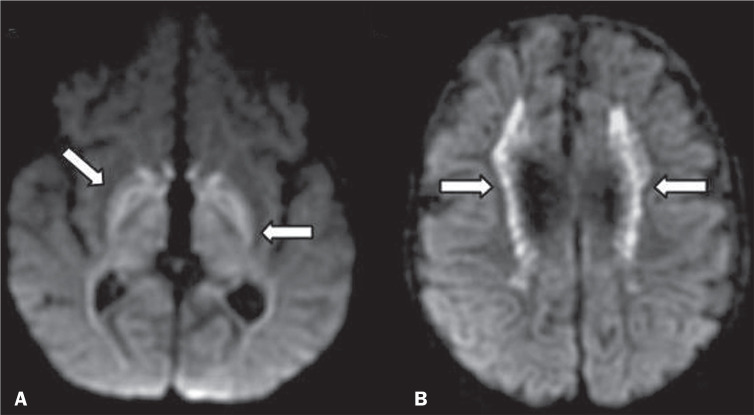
Restricted diffusion in INMDs. Axial acquisitions by DWI. A: Restricted
